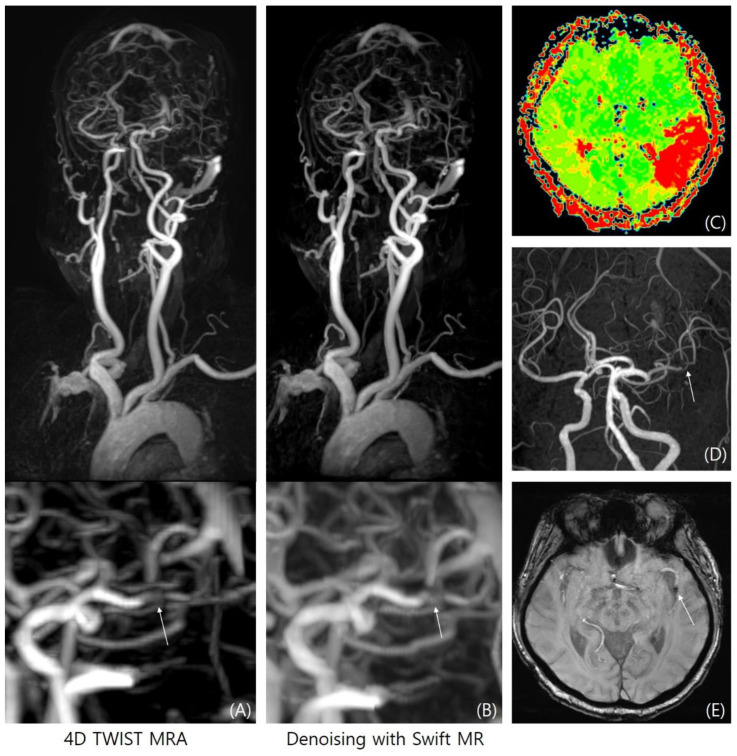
Large vessel occlusion detection. Four-dimensional TWIST-MRA (A) and 4D-DNR (B) showed filling defect at the left distal middle cerebral artery (arrows). Subsequent perfusion MRI (C), TOF-MRA (D), and susceptibility weighted image (E) confirmed thrombus (blooming artifact, arrow) with territorial perfusion delay.
